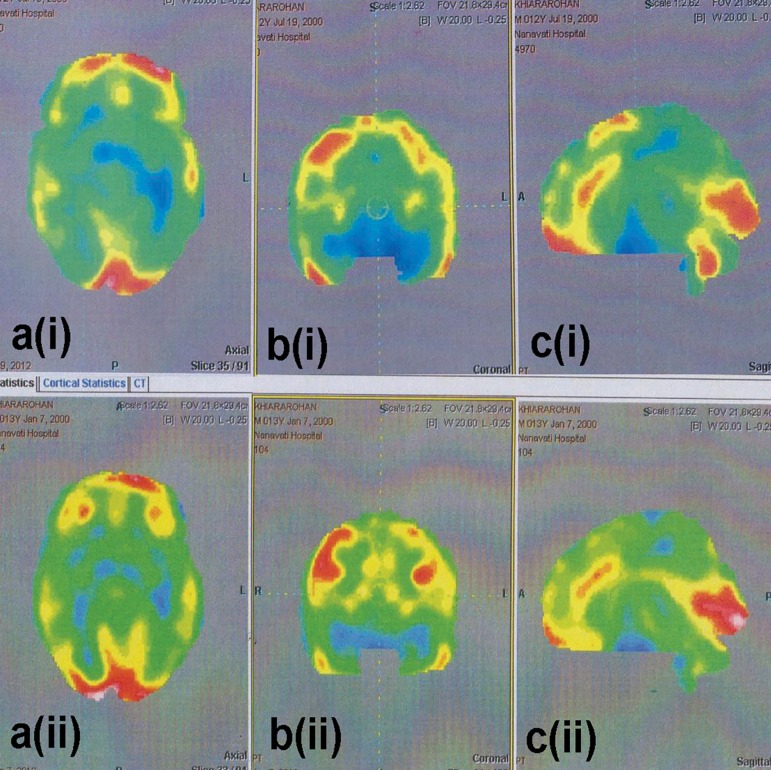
PET-CT scan images obtained before intervention [a(i), b(i), c(i)] and six months after intervention [a(ii), b(ii), c(ii)]. In a(i), b(i), and c(i), blue areas indicate hypometabolism. In a(ii), b(ii) and c(ii), these areas have decreased and turned green which is indicative of normal metabolism. PET-CT: Positron emission tomography- computed tomography.
